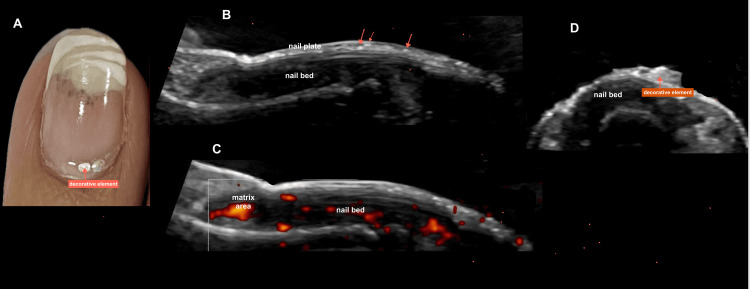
Pseudopsoriasis in chronic gel polish use: clinical and imaging correlation. Female patient, 59 years old, with continuous use of gel nail polish for two years. (A) Clinical image showing a gel manicure with decorative elements adhered to the nail plate (red arrow), along with onycholysis and splinter hemorrhages. (B) Longitudinal grayscale ultrasound image demonstrating loss of the trilaminar pattern of the nail lamina, with irregular, horizontally oriented hyperechoic lines. Red arrows indicate punctiform hyperechoic foci corresponding to methacrylate deposits within the lamina. (C) Longitudinal power Doppler image showing a mild increase in vascularization in the matrix area, indicating inflammatory activity. (D) Axial grayscale ultrasound image with a red arrow pointing to the decorative element adhered to the nail plate. The clinical and imaging findings are consistent with pseudopsoriasis.
